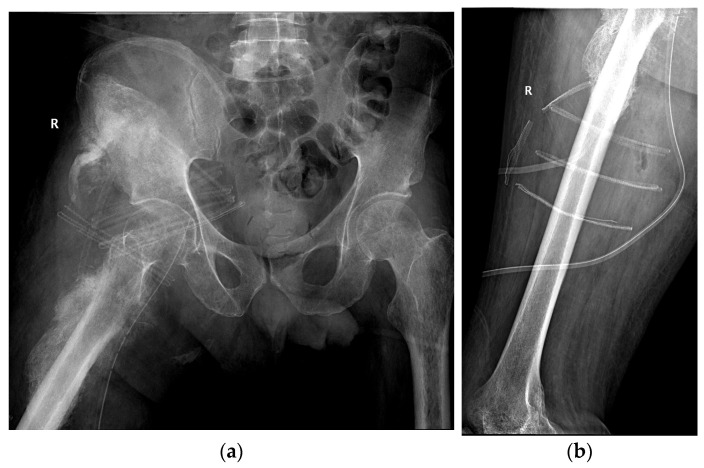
Postoperative radiographs: (a) Lowenstein view of the proximal femur; (b) lateral view of the femur (R—right side).
